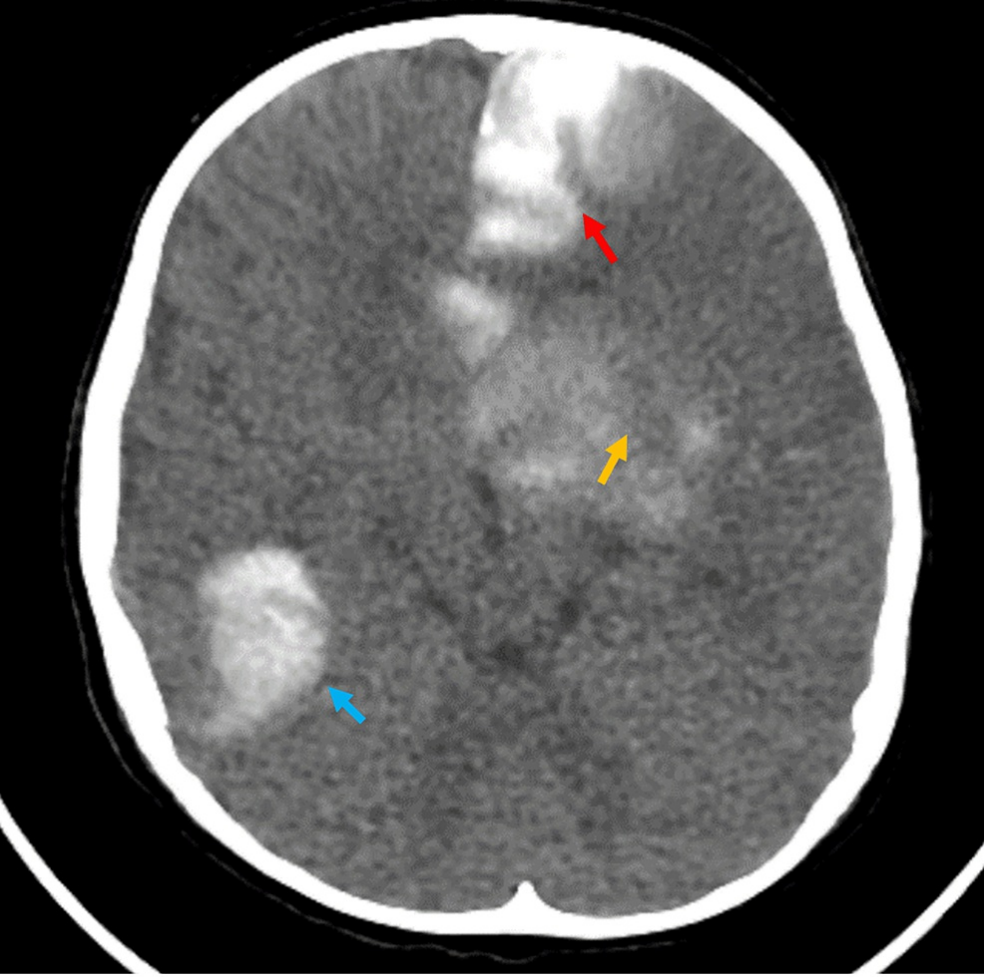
CT of the brain Bilateral intraparenchymal hyperdensities seen in the left frontal extending to the corpus callosum and right temporal lobe (red and blue arrows, respectively) that likely represent contrast extravasation secondary to the previous procedure. Left frontoparietal lobe acute infarction (yellow arrow) with 0.3 cm midline shift to the right side. Blood density in the left basal ganglia that could represent hemorrhagic transformation. CT: computed tomography
